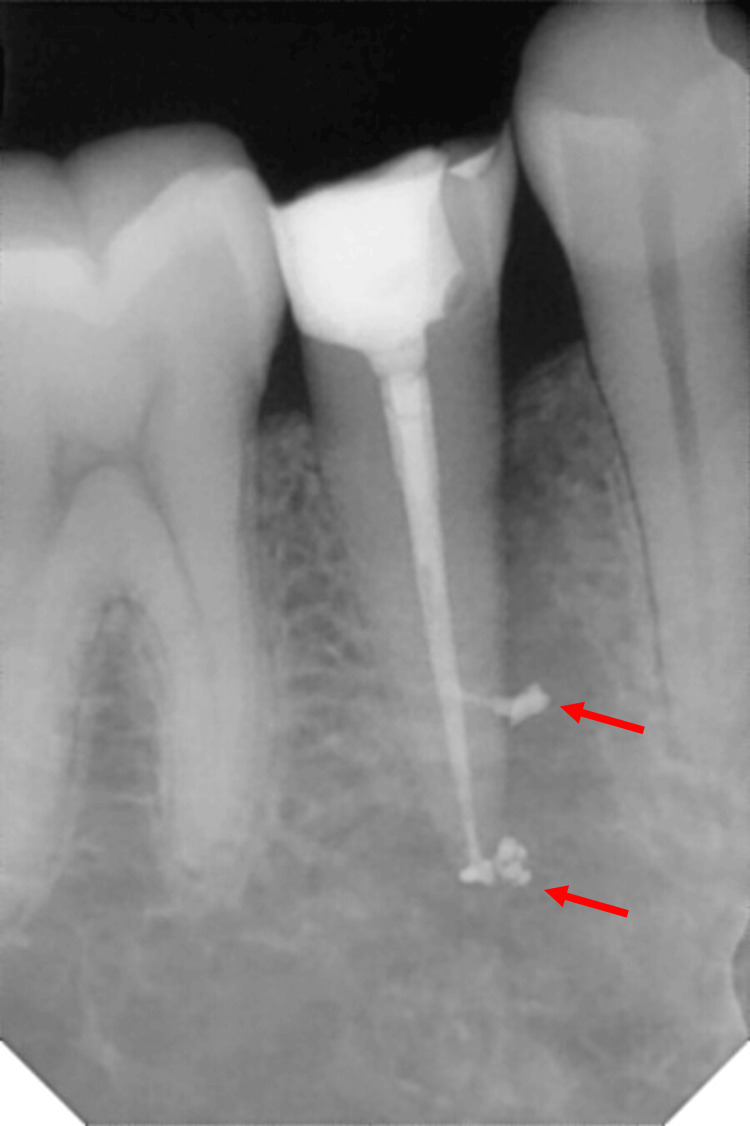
A case showing unintentional apical extrusion of sealer into the periapical region.
